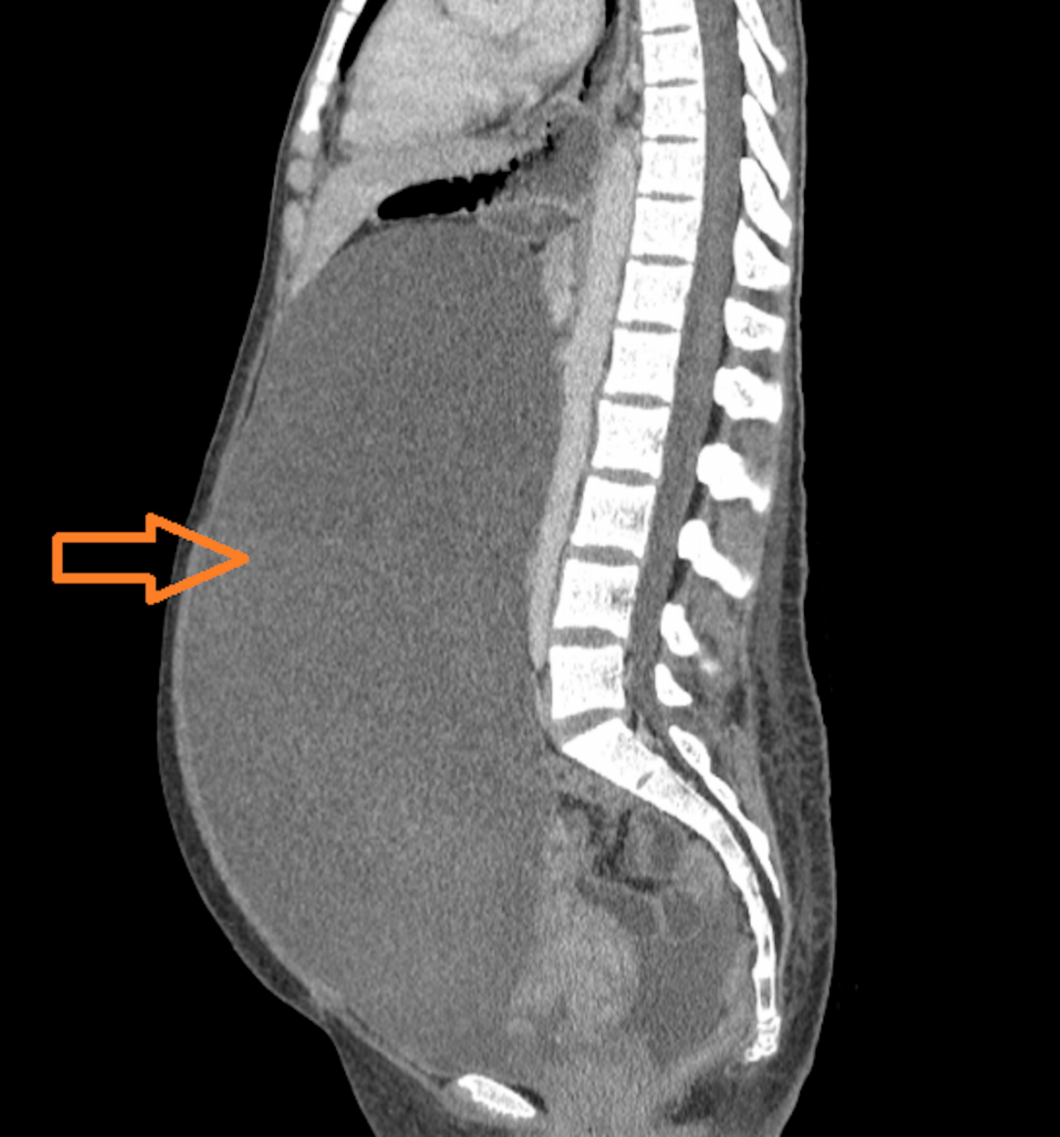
A sagittal CT scan (abdomen and pelvis) depicting an exorbitant mass (arrow) compressing the surrounding abdominal structures.
